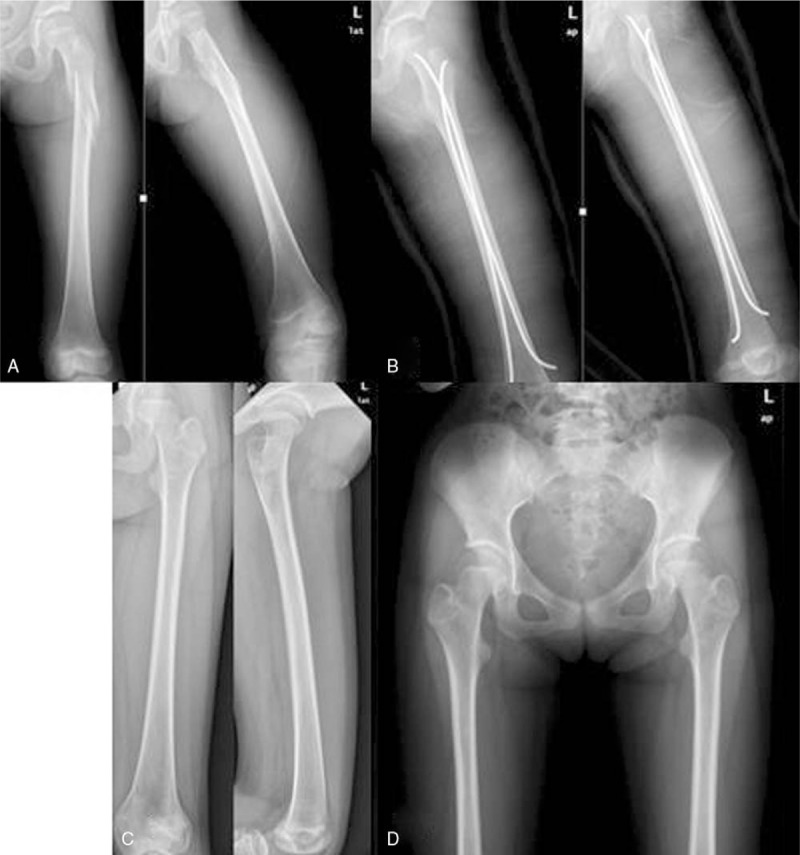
A 7.5-year-old male fell down from a height of 1 m. (A) AP and lateral radiographs of the left femur with long oblique unstable subtrochanteric fracture. (B) Immediate postoperative radiographs after closed reduction and fixation with TENs. (C) Six months after surgery, the fracture had good healing radiographically. (D) AP view of pelvis showed a symmetric normal NSA between involved and uninvolved limb after 38 months.
